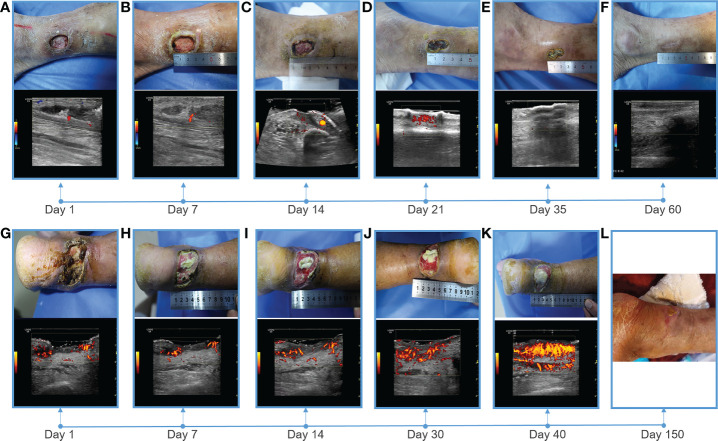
Showing of skin wounds and CDFI or PDI ((Power Doppler Imaging)) ultrasound imaging for progress in the treatment of diabetic lower extremity ulcers in two cases. Wound conditions at the (A) 1th, (B) 7th, (C) 14th, (D) 21th, (E) 35th, and (F) 60th day of post therapy showed the gradual healing of ulceration for case 1; Wound conditions at the (G) 1th, (H) 7th, (I) 14th, (J) 30th, (K) 40th, and (L) 150th day of post therapy showed the gradual healing of ulceration for case 2. PDI showed that the blood flow of the soft tissue around the ulcer gradually increased, and the blood flow was very abundant before healing.
